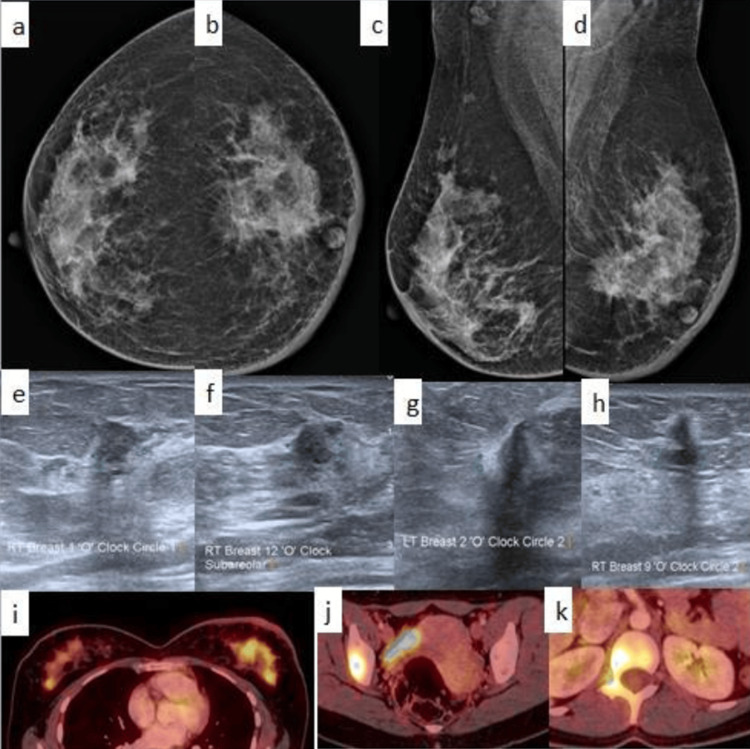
A case of a 29-year-old lactating female with pregnancy-associated breast cancer a-d: bilateral CC and MLO views on X-ray mammogram show lesions in both breasts with partially obscured margins and surrounding architectural distortion; e-h: ultrasound shows multiple bilateral irregular lesions with angular margins; i-k: FDG PET shows bony metastases with FDG-avid lesions in both breasts, the right iliac bone, and the vertebra. CC: craniocaudal; MLO: mediolateral oblique; FDG: fluorodeoxyglucose
